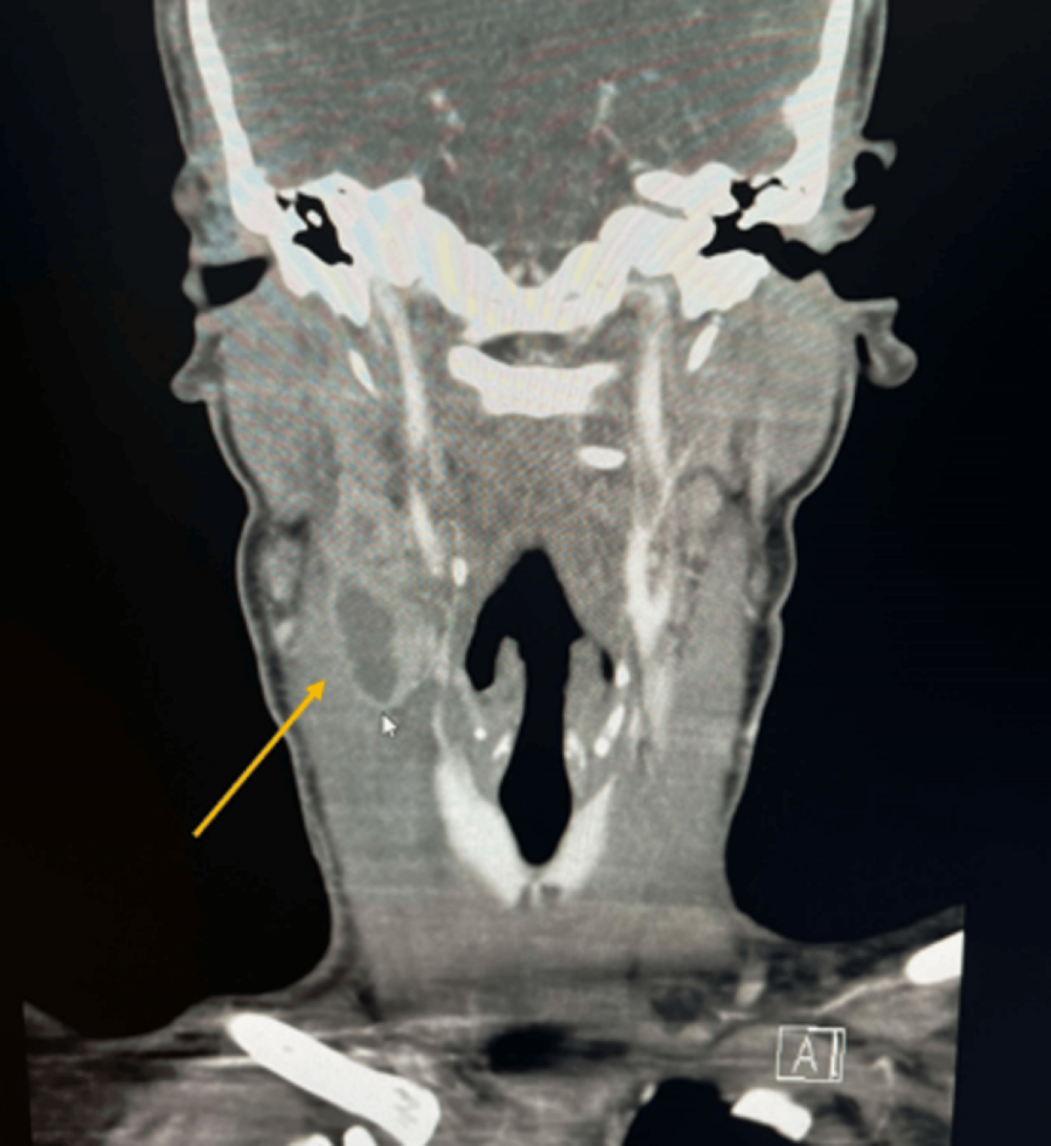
Initial CT on admission showing a necrotic mass measuring 2.4 x 1.7 cm in the right level 2 cervical chain with adjacent sternocleidomastoid myositis and mass effect on the internal jugular vein.
